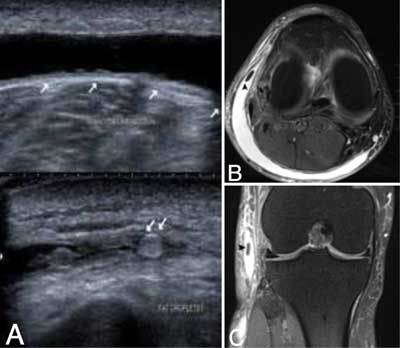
Fat suppressed (FS) T2-weighted (WI) MRI of the right knee revealed a half-moon shaped hyperintense collection between the subcutaneous fat and the fascia of the calf muscle and lateral collateral ligament (Fig. B, axial FS T2-WI). A small focal area of isointense signal compared to subcutaneous fat was seen within the lesion (Fig. B, axial FS T2-WI and Fig. C, coronal FS T2-WI, black arrowheads), in keeping with a fat globule. The diagnosis of Morel-Lavallée lesions was made.
